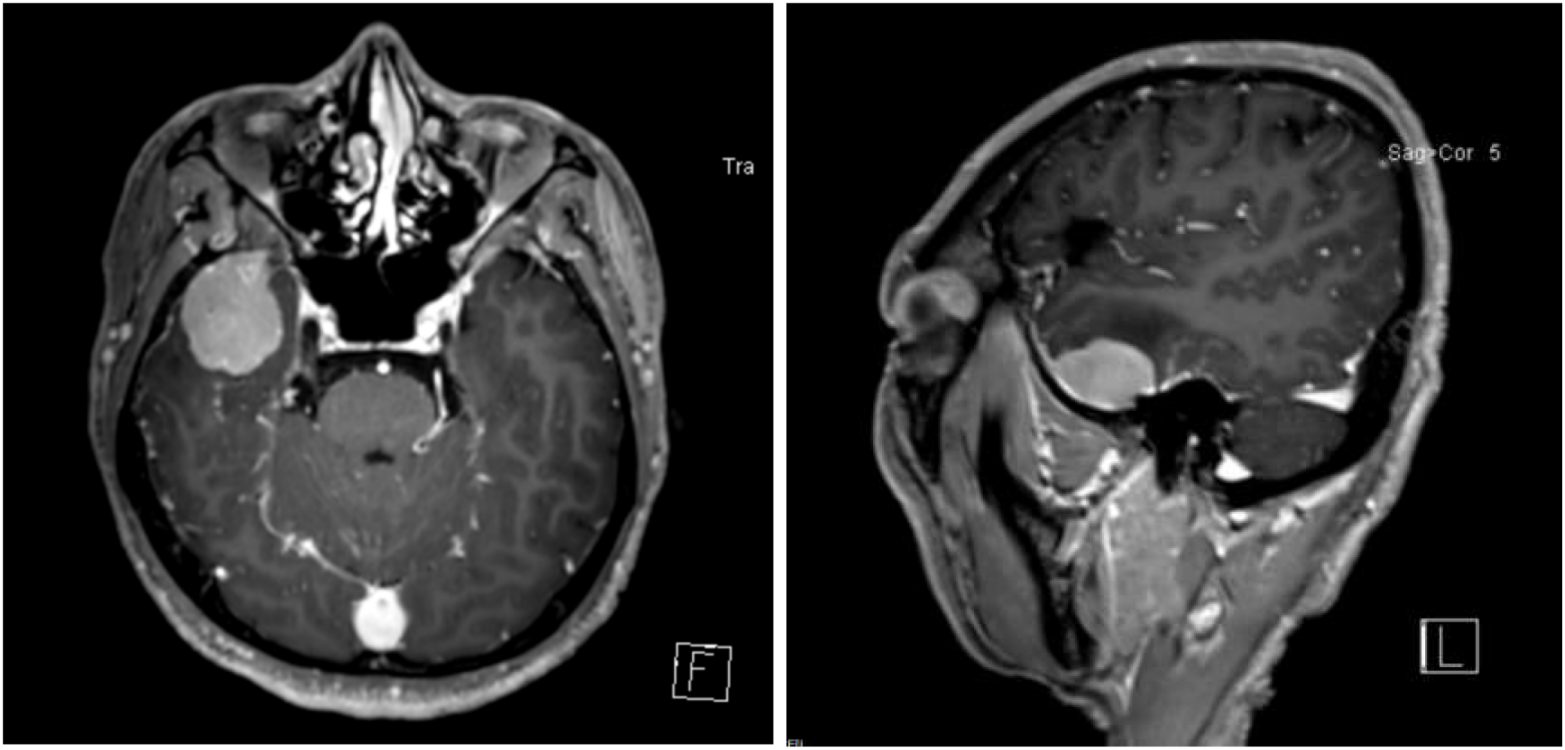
Contrast-enhanced cranial MRI demonstrating a space-occupying lesion in the right temporal region.
